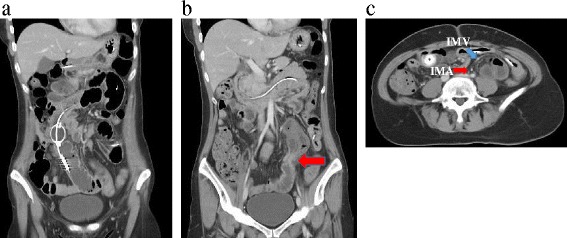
a CT shows dilation of the small bowel on the oral side of the obstruction. b Obstruction (*arrow*) with a closed loop of the small bowel. c The incarcerated small bowel was successively covered by sigmoid mesocolon
